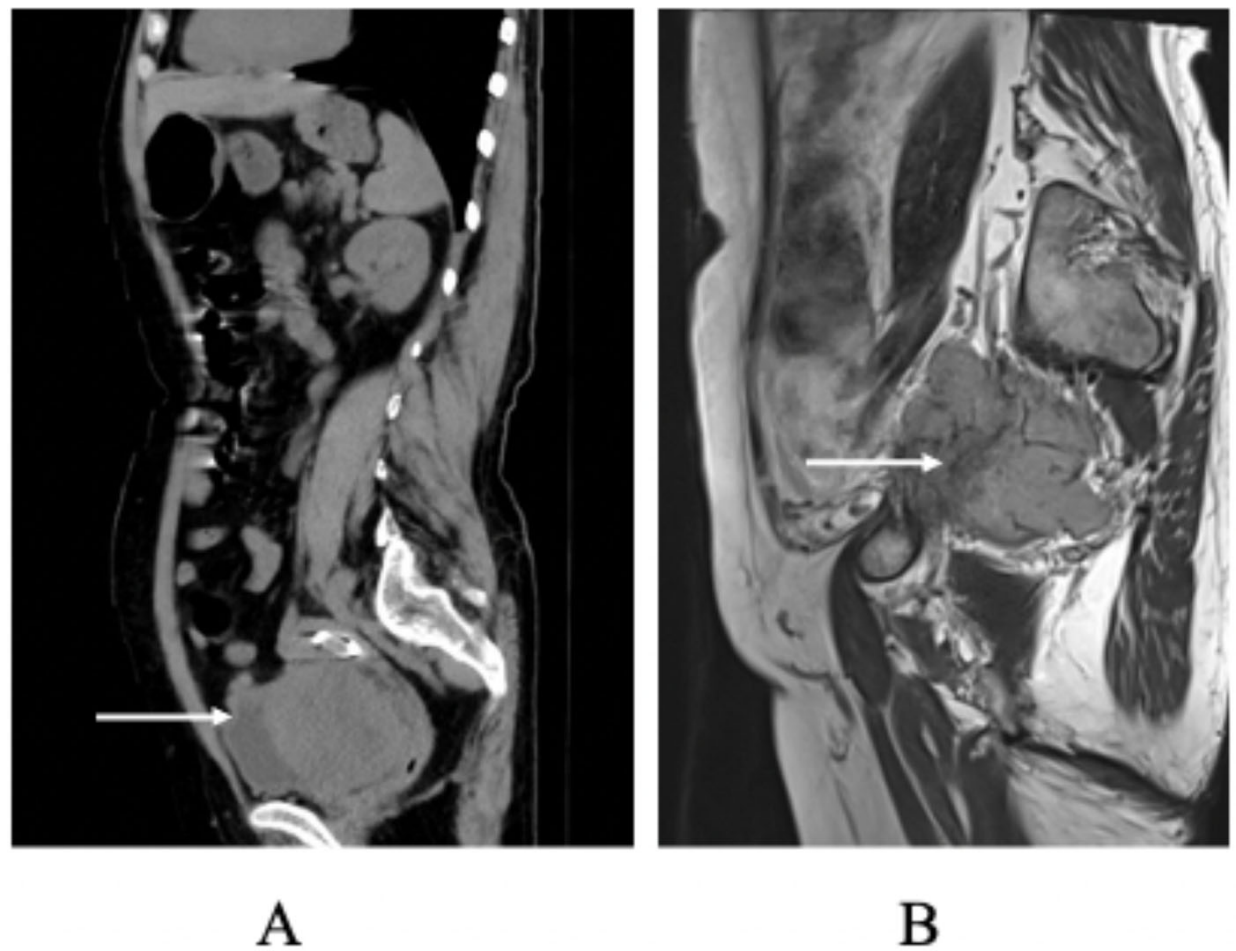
(A) Preoperative Non-contrast Abdominal CT Scan: this image depicts the non-contrast abdominal CT scan of the patient obtained before surgical intervention. (B) Postoperative MRI Scan at One Month: MRI was performed one month postoperatively to consider tumor recurrence.
